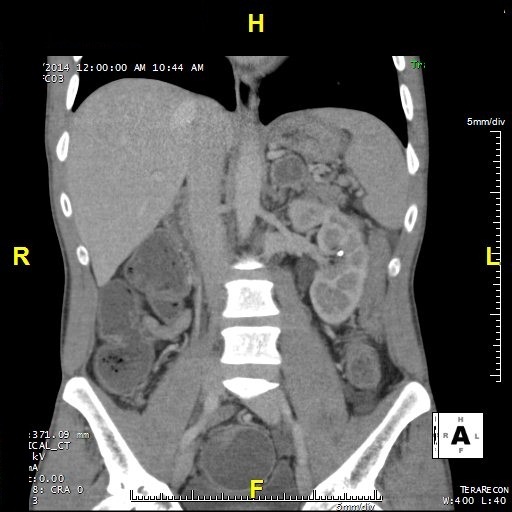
CT scan longitudinal view pancreatic cyst located in the tail of pancreas
